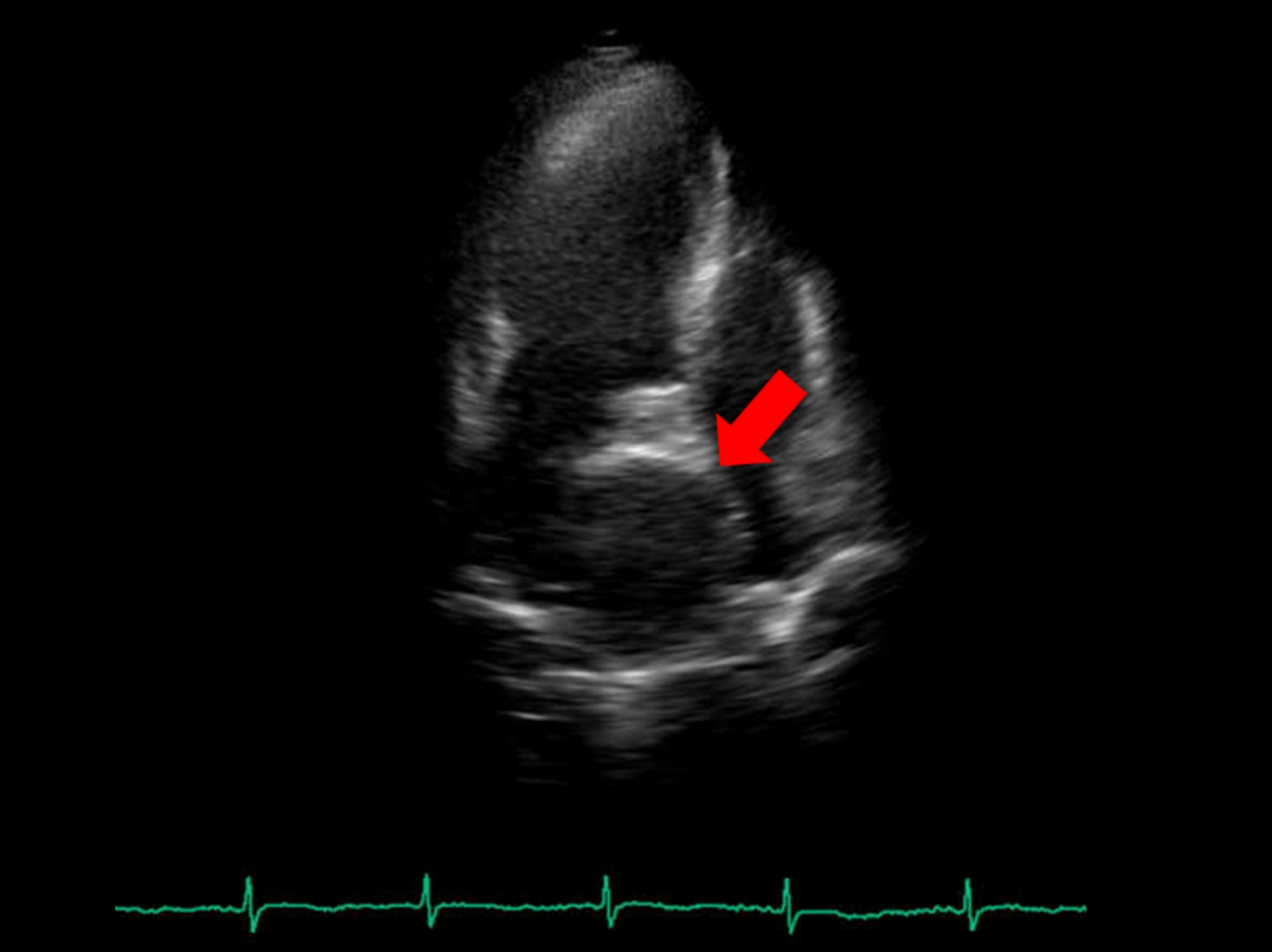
Echocardiogram before surgery. Transthoracic echocardiogram showing a round-shaped hypoechoic lesion (red arrow) of 62 mm diameter between right and left atria.
